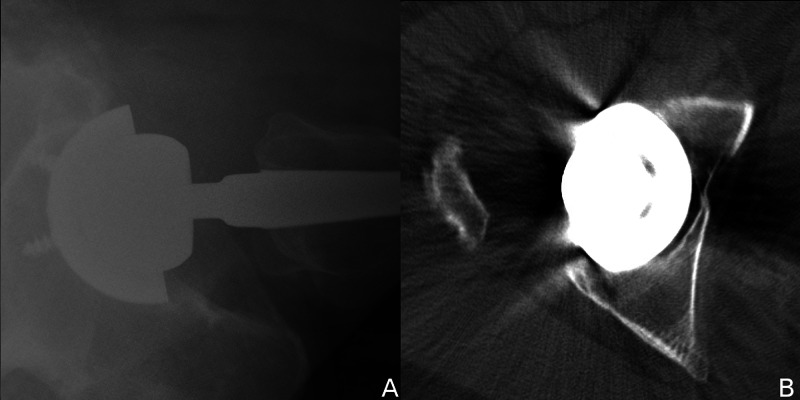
Radiographic diagnosis of IPI. (A) Cross-table lateral radiograph with anterior cup prominence from relative retroversion. (B) Axial CT scan of the right hip with anterior rim of the cup extending beyond the anterior wall of the acetabulum. IPI, iliopsoas impingement; CT, computed tomography
